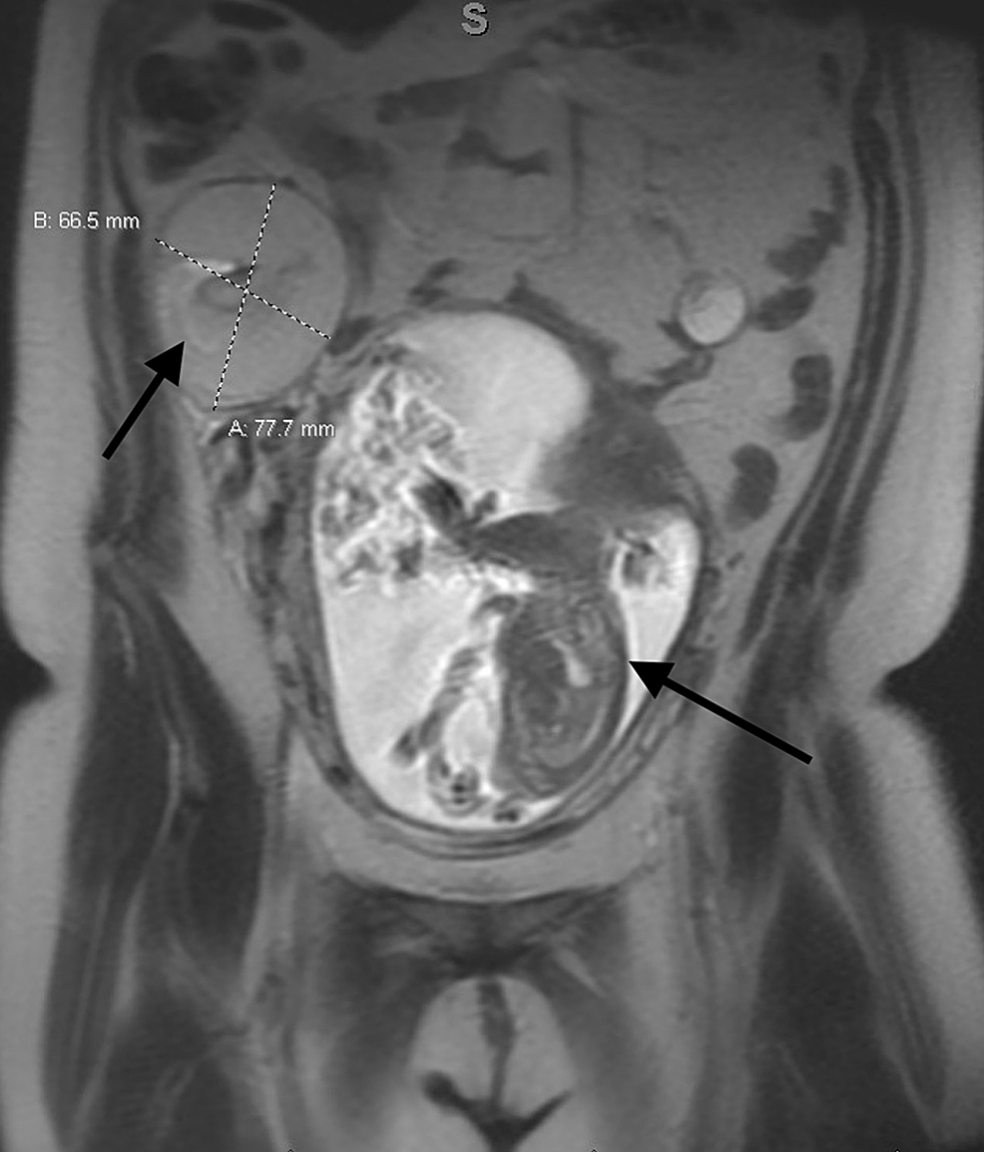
Coronal MRI showing a 7.7 cm x 6.6 cm right adnexal mass along with intrauterine pregnancy.
